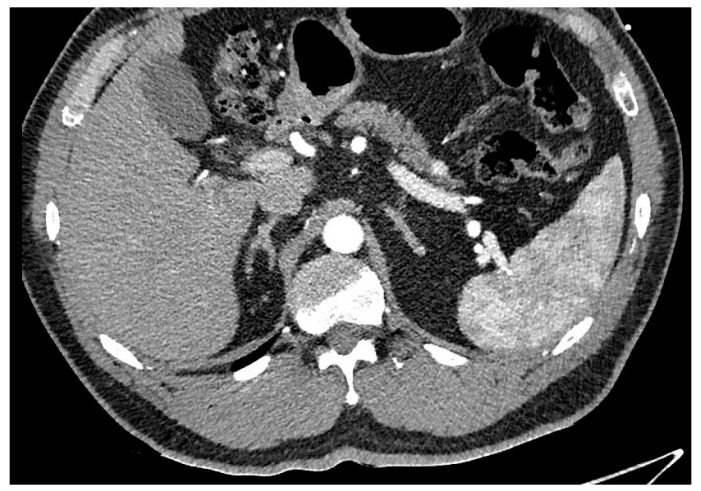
CT arterial phase with lesion in the tail of the pancreas consistent with neuroendocrine tumour.
